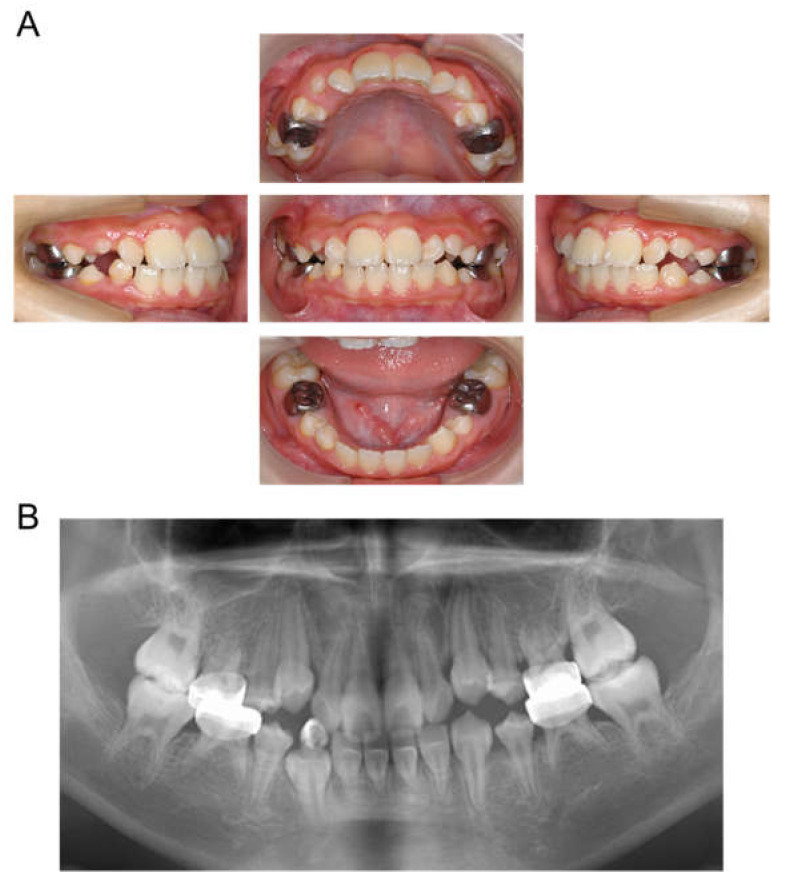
Images at the age of 10 years and 2 months. (A) Intraoral photographs. (B) Panoramic radiograph.
